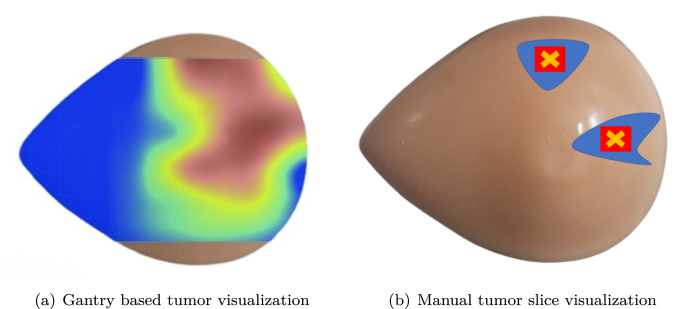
Comparison of slice prediction using the angular ultrasound gantry (a) and manual method (b). The gantry provides tumor probability for each slice, aiding accurate tissue selection, while manual prediction is time-consuming, error-prone, and lacks quantitative guidance.
